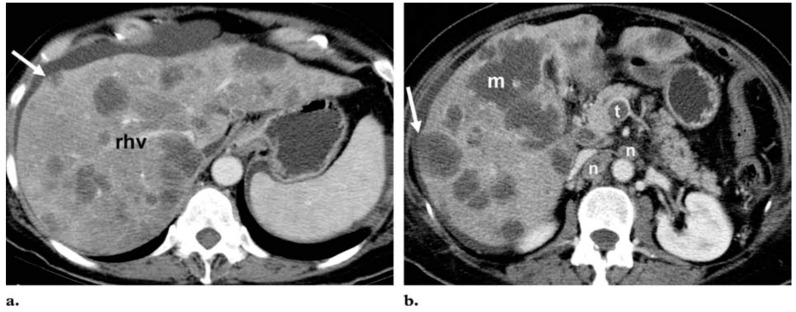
Differentiation of metastases from fat deposition in the liver. Axial portal venous phase contrast-enhanced CT images at the level of the right hepatic vein *(rhv)* (a) and the pancreatic head (b) show innumerable hypoattenuated lesions throughout the liver. Most of the lesions are round or oval, but the largest (*m* in b) has a geographic configuration. Because of their low attenuation (<40 HU), the lesions might be mistaken for multifocal fat deposition; however, the mass effect of the lesions, which produces bulging of the liver surface (arrow) and compression of the right hepatic vein, as well as the multiplicity of lesions, their predominant round or oval shape, the thrombus (*t* in b) in the superior mesenteric vein, and numerous heterogeneous lymph nodes (*n* in b), are suggestive of malignancy. The lesions were identified as hematogenous metastases from pancreatic adenocarcinoma. Figure reproduced with permission from Hamer et al. [55].
